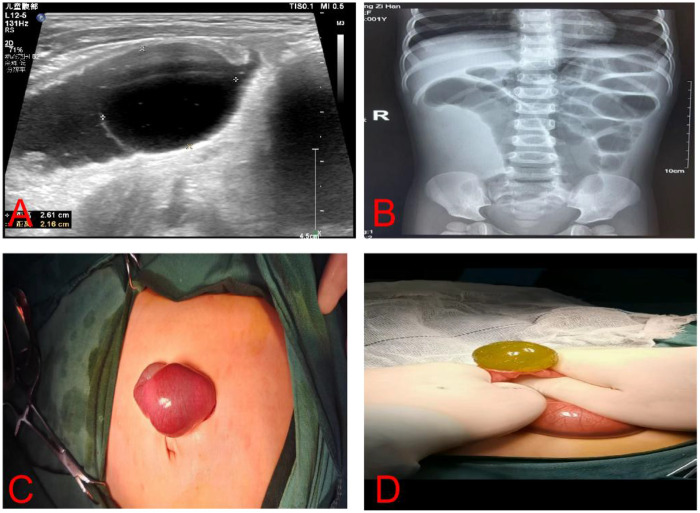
Imaging findings and intraoperative observations of intestinal obstruction caused by a hydrogel bead (female child, 1 year old). (A) Ultrasound shows an anechoic area within the intestinal lumen in the right lower abdomen with good acoustic transmission. (B) Abdominal x-ray shows no visible foreign body; intestinal gas is present with uneven distribution, along with localized fluid accumulation and air–fluid levels. (C) Intraoperative findings show localized dilation of the ileum with a foreign body inside. (D) Surgical incision of the intestinal wall reveals a yellow hydrogel bead.
